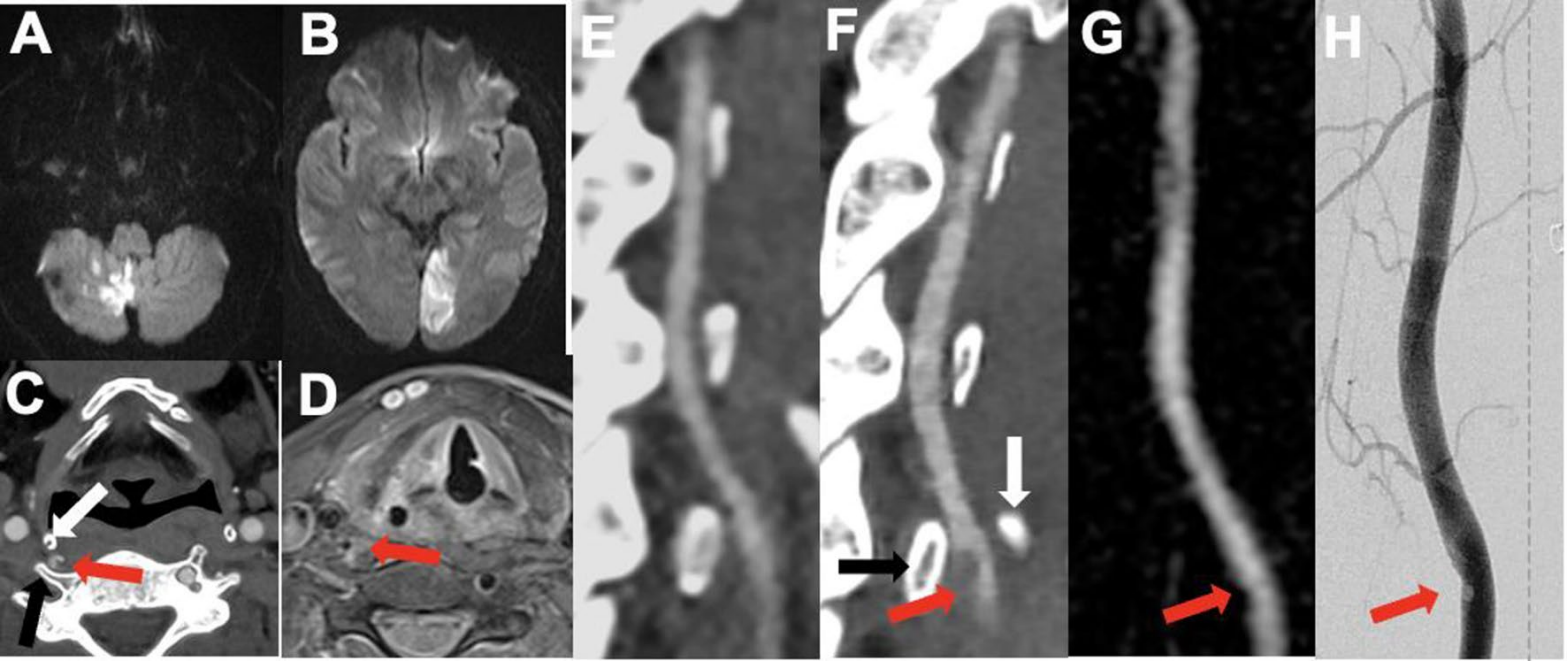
Brain MRI diffusion-weighted images depict right PICA infarct on d0 (A) and left PCA infarct on d25 (B). C CT angiogram shows compression of aVA-C4 at d25 between the superior cornu of the thyroid cartilage (white arrow) and the C5 transverse process (black arrow). A crescent-filling defect is depicted with a red arrow. D No signal abnormalities were observed in fat-saturated T2w sequences at d30. A red arrow depicts aVA-C4. E CT angiogram on d0 does not show luminal irregularity. F Corresponding sagittal reformation to the axial images depicted in (C). On day 30, the filling defect was reduced on MR angiography (G) and in digital subtraction angiogram (H)
